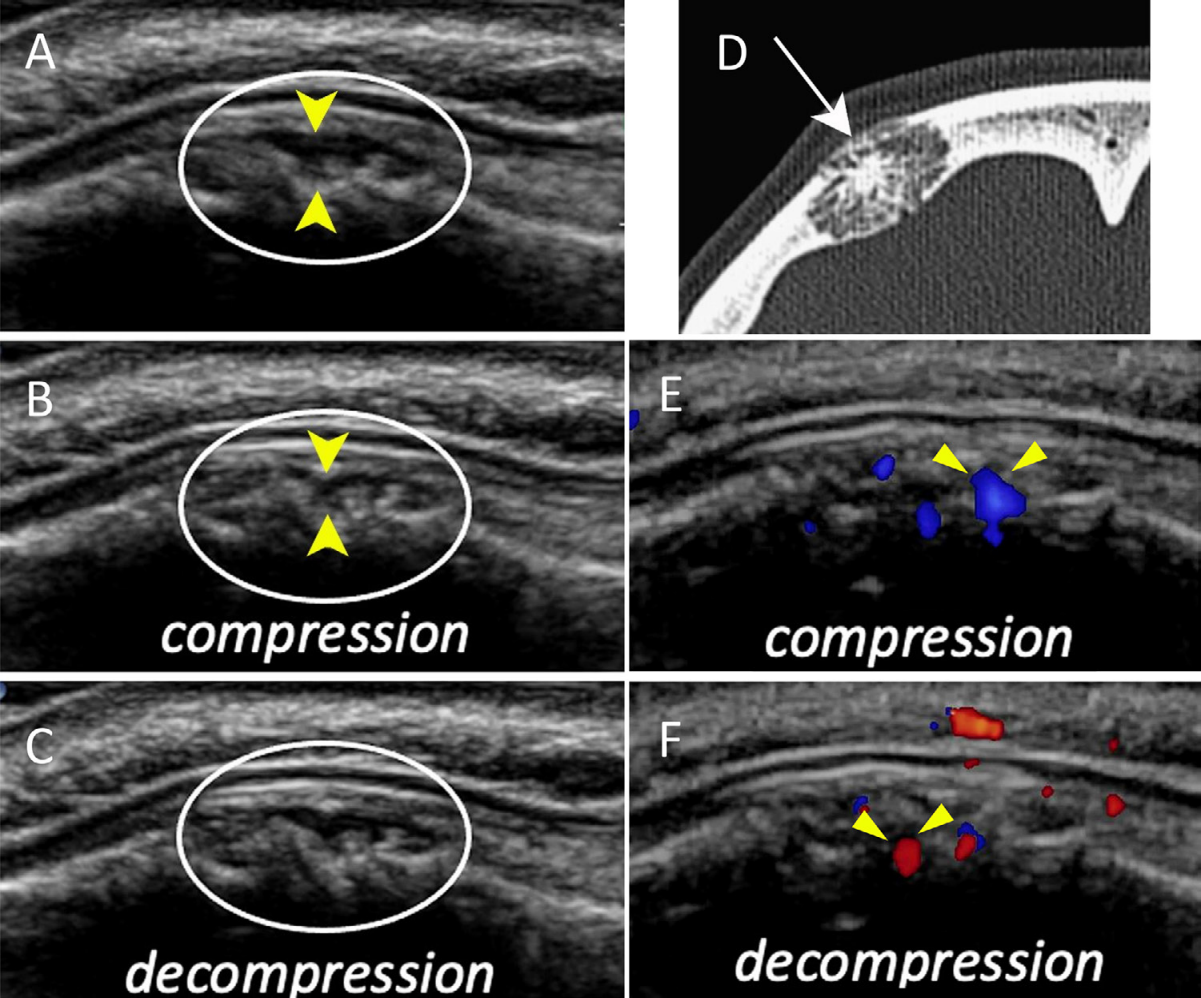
Calvarial venous malformation of the right frontal bone. A: Ultrasound image showing hypoechoic thickening of the right frontal calvarium with irregular cortical surface (yellow arrowheads). B, C: Ultrasound during compression (B) and decompression (C) demonstrating compressible cavities (yellow arrowheads). D: CT image showing an expansile osteolytic lesion of the right frontal bone with radiating trabeculae, creating a sunburst/honeycomb appearance. E, F: Color Doppler ultrasound during compression (E) and decompression (F) demonstrating a flow–reflux phenomenon with inversion of flow color during ther maneuver (arrowheads).
